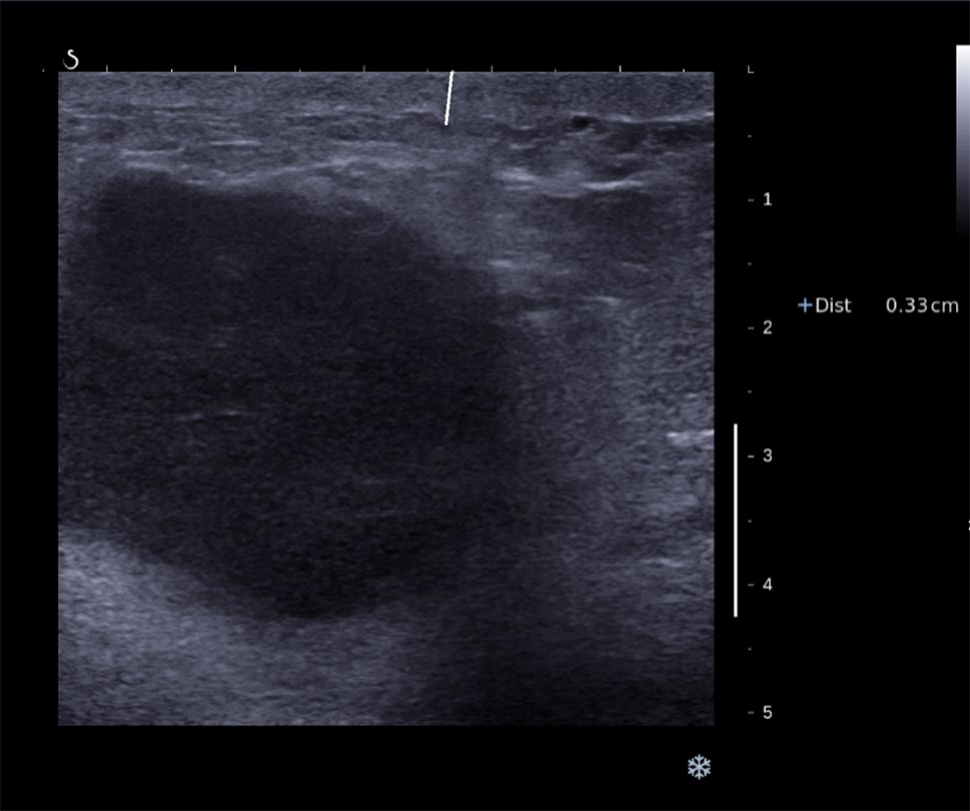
Ultrasound image of a triple negative breast cancer showing thickening of the overlying skin as indicated by the callipers
